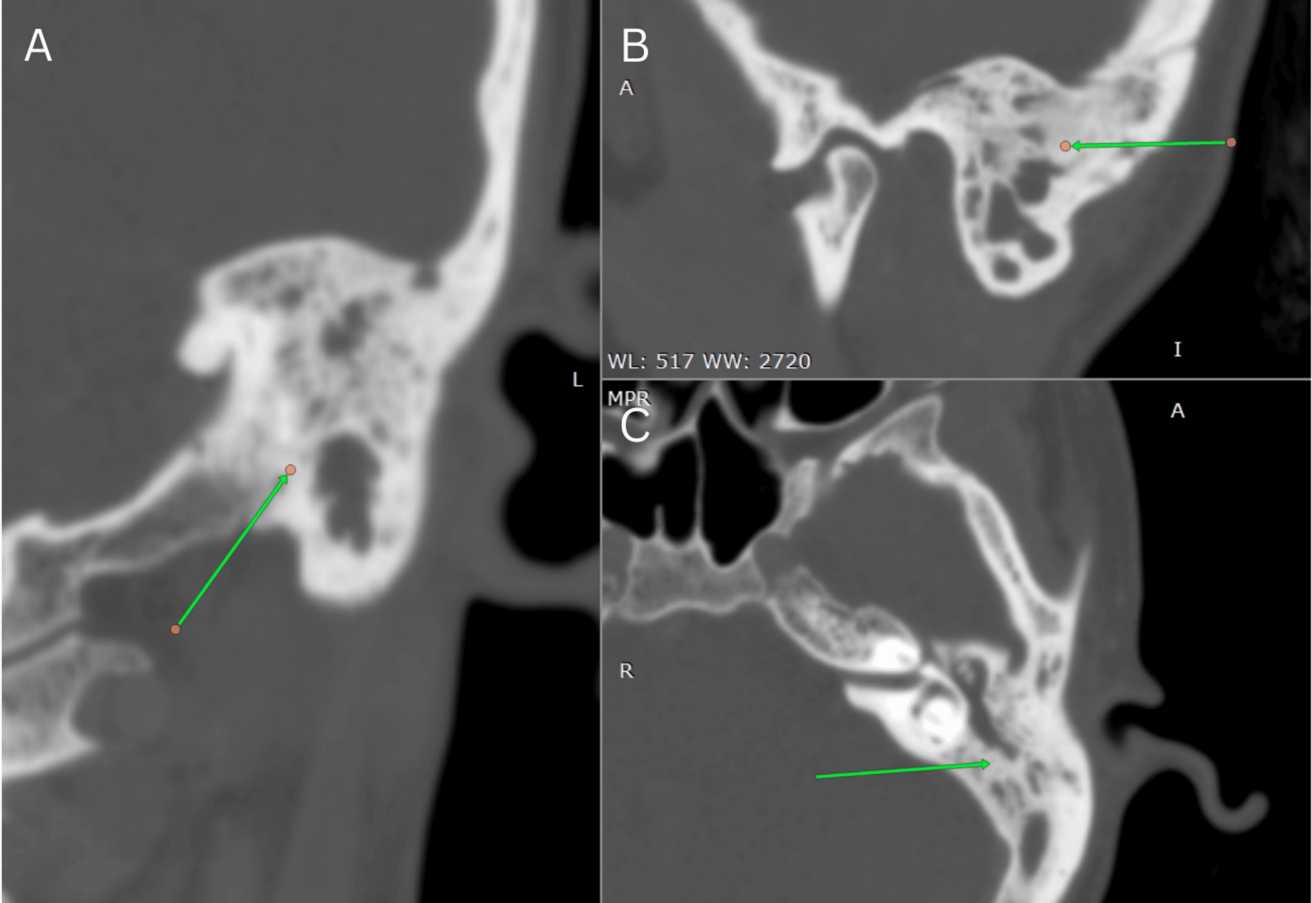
High-resolution computed tomography of the left temporal bone (April 2025) (A) Sagittal/oblique view (coronal reformat): complete soft tissue opacification of the left external auditory canal and middle ear cleft (green arrows), consistent with otomastoiditis. (B) Axial view: dense inflammatory soft tissue filling the mastoid air cells (green arrow), consistent with otomastoiditis. (C) Coronal view: soft tissue within the middle ear and mastoid antrum abuts the tegmen tympani (mastoid roof) with preserved bony margins.
